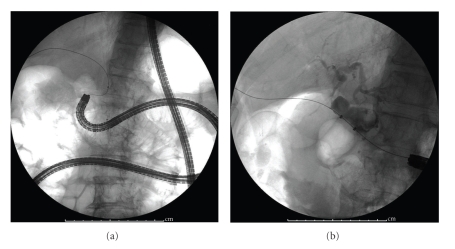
Enteroscope assisted ERCP. (a) X-ray film showed that an enteroscope reached the papilla using rendezvous technique. (b) Cholangiogram revealed bile duct stones.
